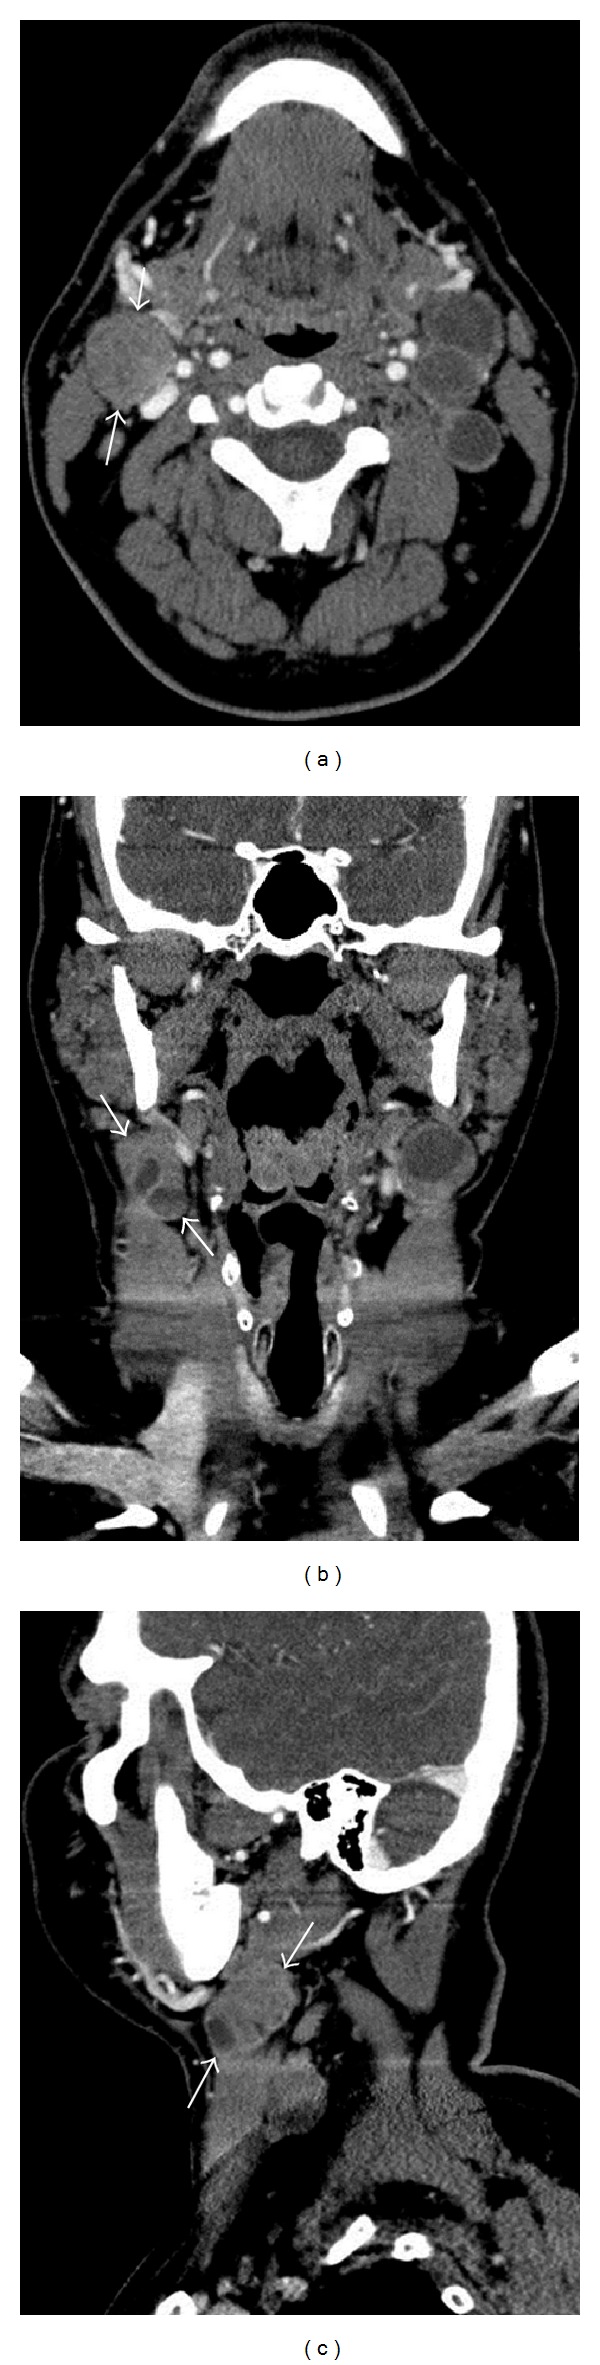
Detection of the largest lymph node in a patient with mucosal-based SCCa and neck lymphadenopathy by CT with IV contrast. Arrows demonstrate the longest axis of a selected “enlarged” lymph node within level IIA on (a) axial, (b) coronal, and (c) sagittal planes. The primary tumor was a left-sided oropharyngeal squamous cell carcinoma (SCCa). The selected lymph node shows partial central necrosis.
